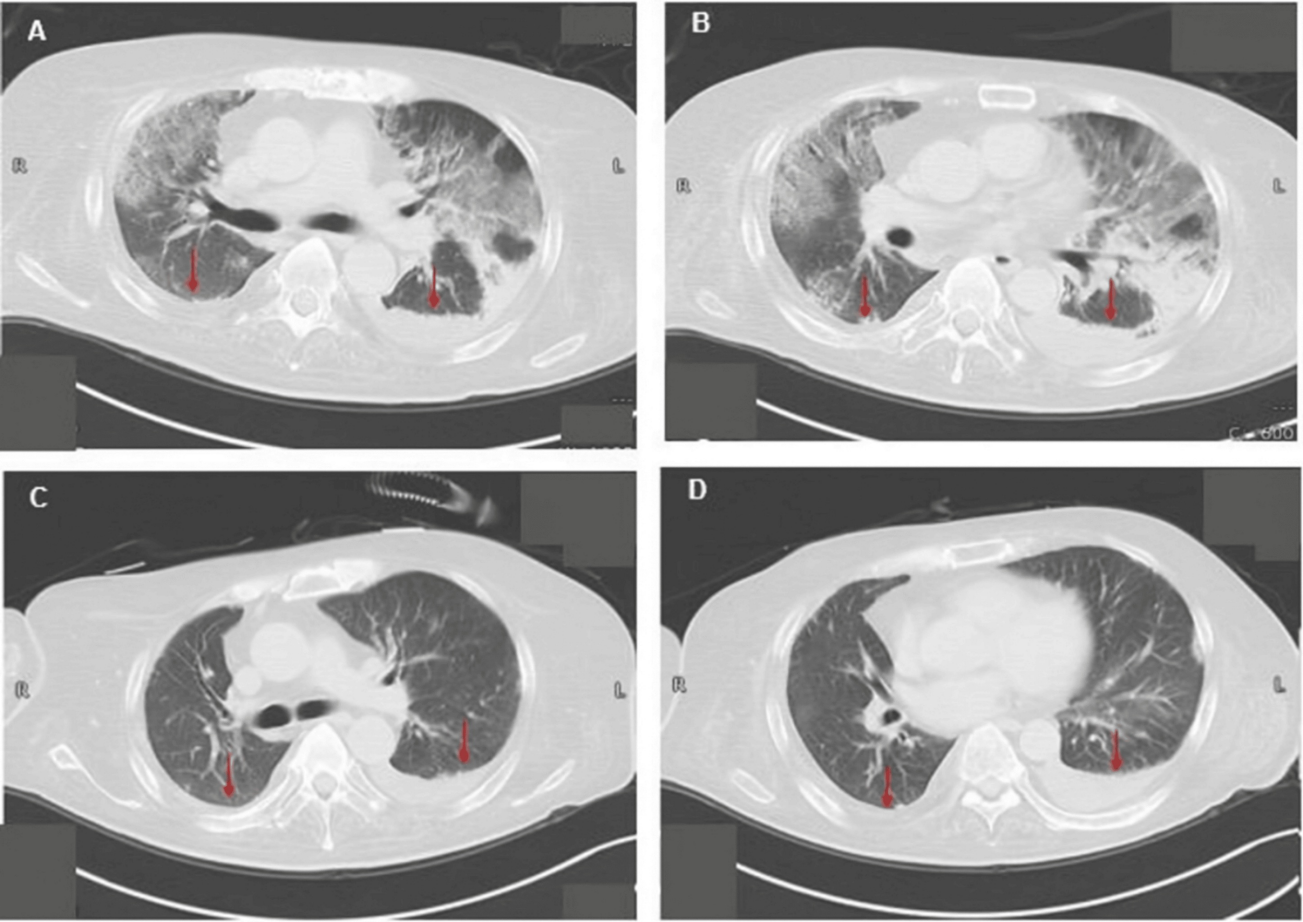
Axial chest CT images on day 0 and day 21. (A) Axial chest CT image obtained on day 0, showing bilateral pulmonary infiltrates predominantly in the posterior regions (arrows).
